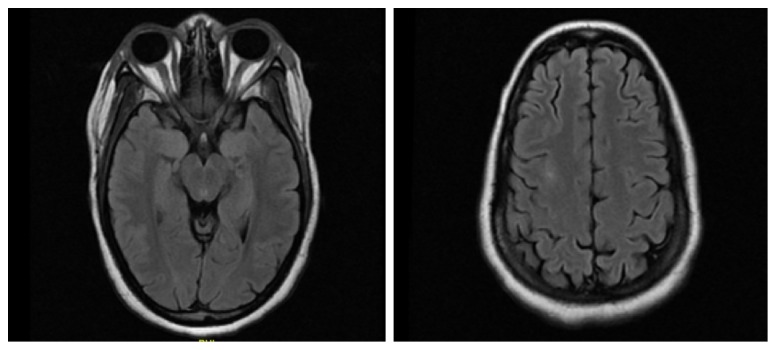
MRI brain without contrast five days later showing resolution of previously seen areas of cortical and subcortical white matter hyperintensities on the FLAIR sequence. *Abbreviation:* MRI, Magnetic Resonance Imaging; FLAIR, Fluid attenuation inversion recovery.
